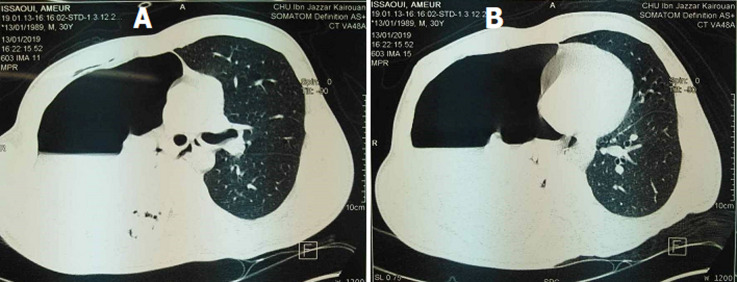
(A,B) thoracic computed tomography (CT) scan showing a large right-sided compressive hydropneumothorax with lung collapse and pleural effusion
